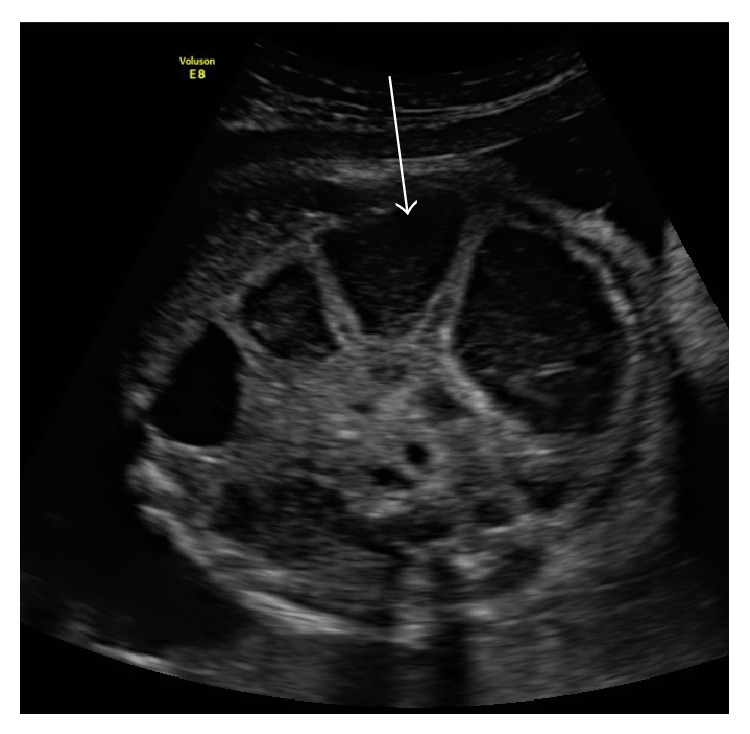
Ultrasound image demonstrating intra-abdominal loops of bowel dilatation in fetal gastroschisis at 33 weeks of gestation.
